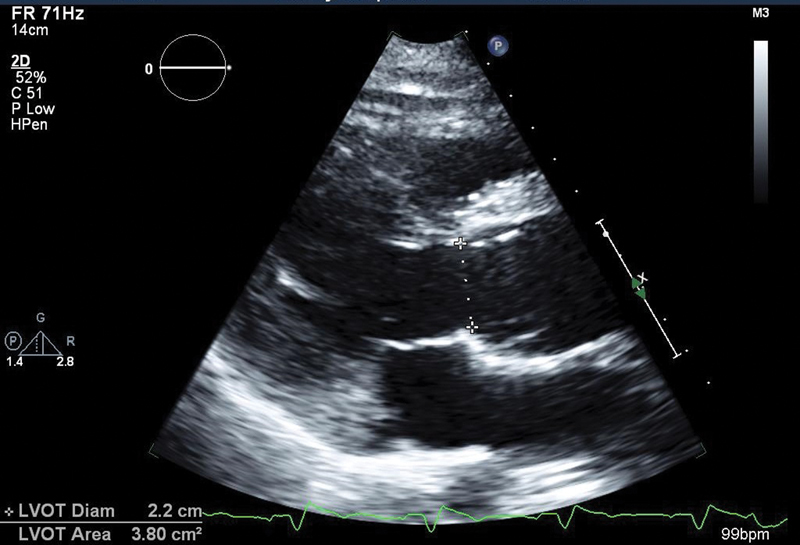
Echocardiogram after the Ross procedure, aortic root.
